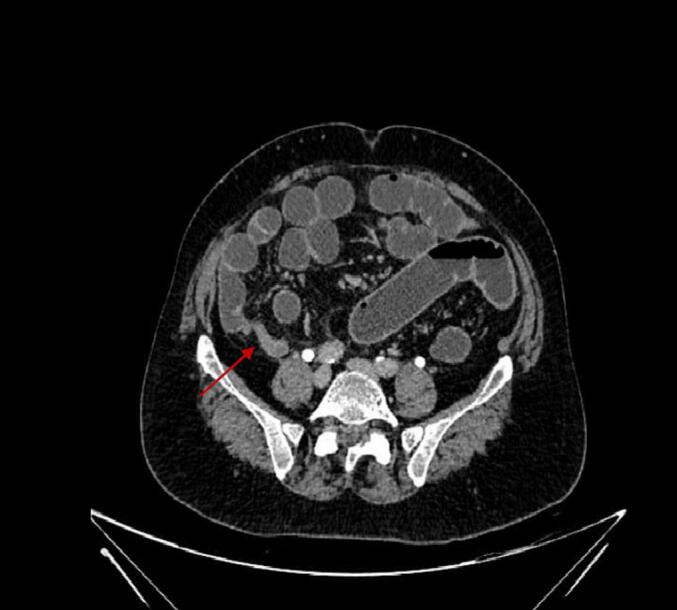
CT appendix body of Patient 2.
